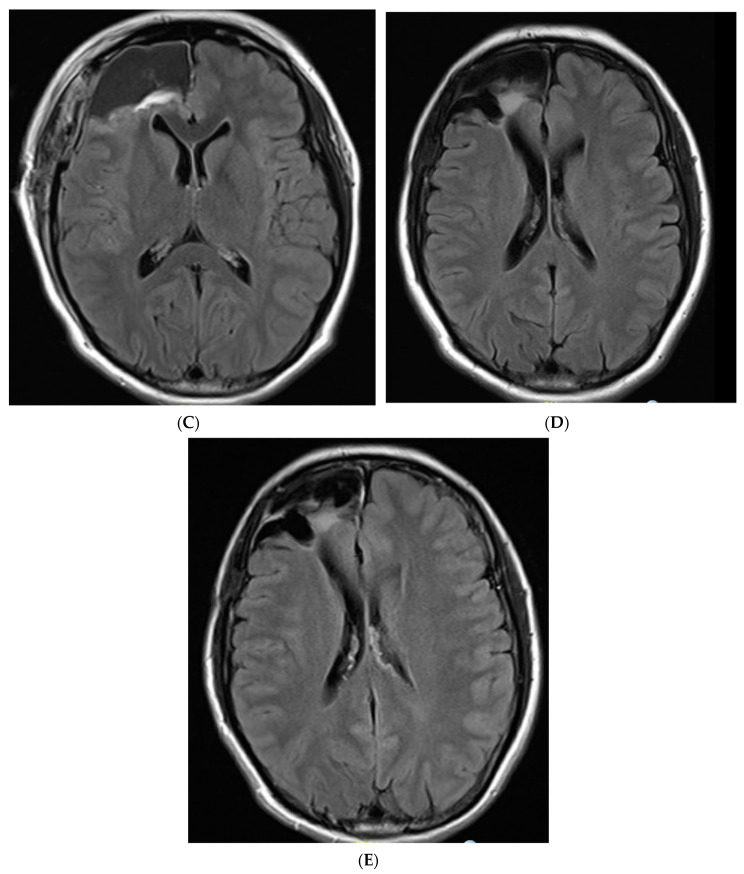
FLAIR sequence axial MRI brain images. (A) 2.6 × 2.0 cm lesion in right frontal lobe (pre-operative). (B) T1 post contrast pre-operative image of right frontal lobe lesion (pre-operative) (C) Hyperintense blood products in resection bed (post-operative day 1), (D) Focal area of increased T2/FLAIR signal deep to resection cavity (prior to starting ICI, 9 months after resection). (E) T2/FLAIR hyperintense tissue changes in right frontal lobe appear stable (18 months post resection).
